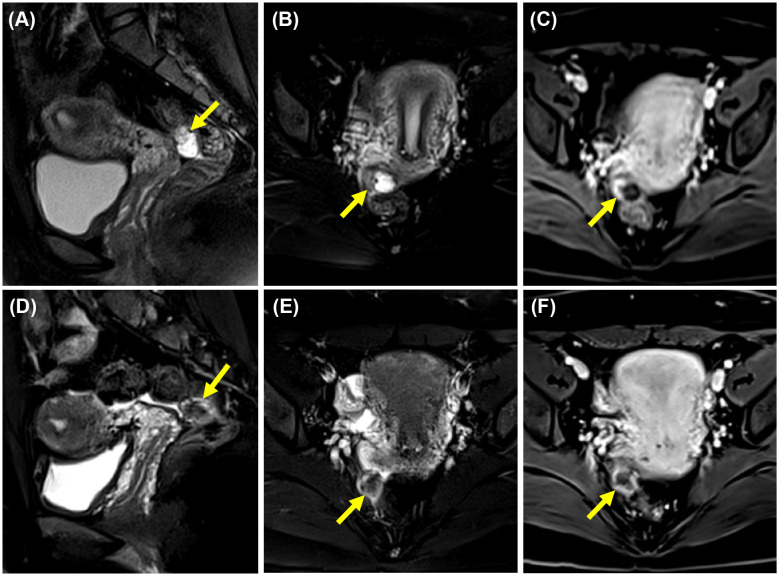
Pelvic MRI findings at the initial presentation and at recurrence. (A–C) Pelvic MRI in 2021 showing a right-sided cystic-solid lesion (1.7 × 1.9 × 1.9 cm) between the cervix and the rectum. On T2-weighted images (A, B), the lesion shows heterogeneous high signal intensity (arrows). On the contrast-enhanced T1-weighted image (C), ring-like peripheral enhancement is observed (arrow). (D–F) Abdominopelvic MRI in 2024 showing a round-like cystic-solid lesion (1.7 × 2.0 × 1.7 cm) at the same location. On T2-weighted images (D, E), the lesion appears heterogeneous with slightly low signal intensity (arrows). On the contrast-enhanced T1-weighted image (F), ring-like peripheral enhancement is again observed (arrow).
